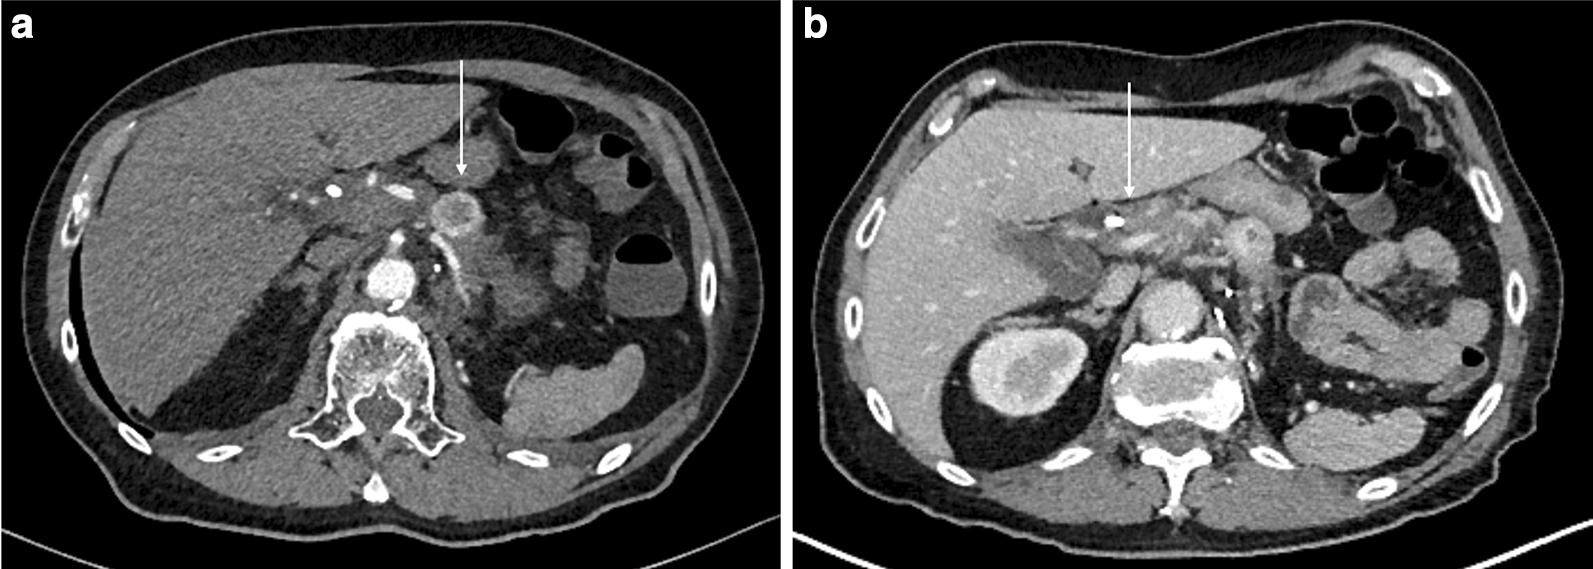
Computed tomography scans of the pancreas. a Hypervascular lesion in the body/tail of the pancreas (arrow), highly suspicious for a renal cell cancer metastasis or neuroendocrine tumor. b Hypodense, more proximally located lesion in the pancreas (arrow), suspicious for adenocarcinoma
